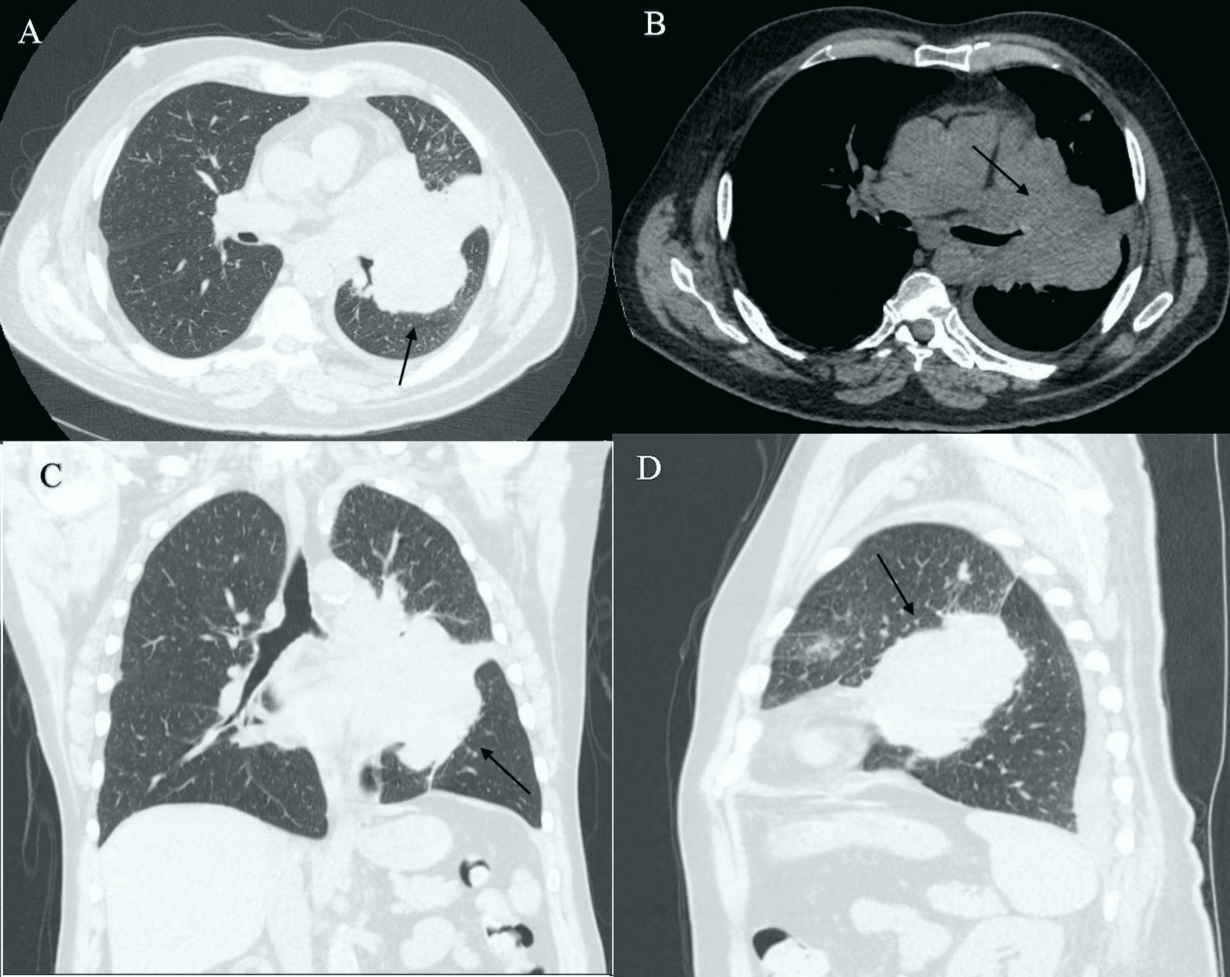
CT chest without contrast before the procedure. (A) Lung window view showing left hilar mass (arrow), (B) mediastinal view demonstrating left hilar adenopathy (arrow), (C) coronal view of left hilar mass (arrow), and (D) sagittal view of left hilar mass (arrow).
